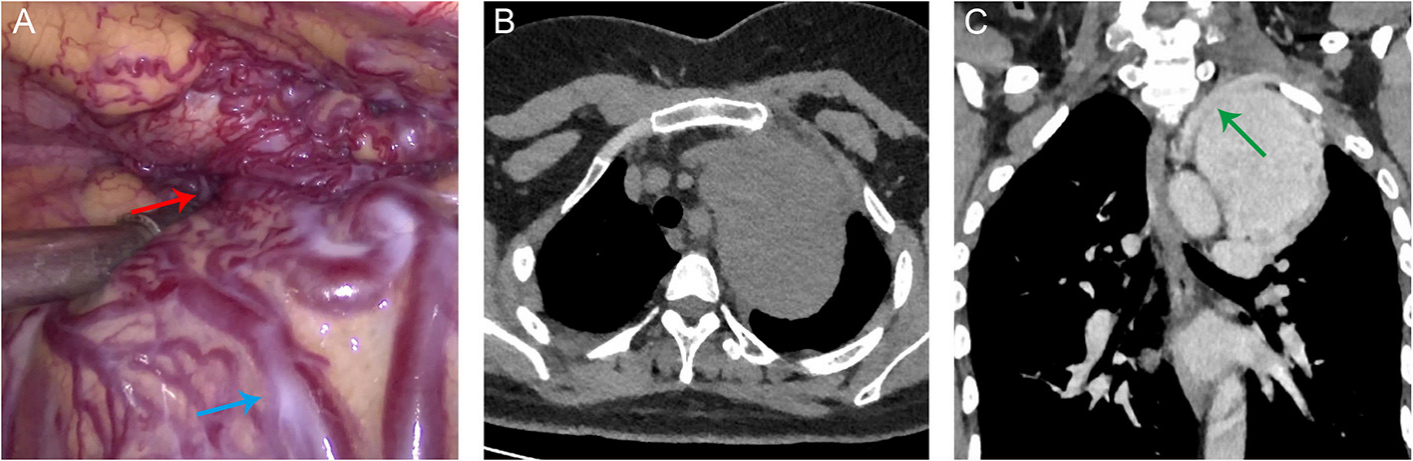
(A) Intraoperative photograph showing abundant tortuous blood vessels on the surface of the tumor (blue arrow) and an unclear border at the top of the chest (red arrow). (B) A CT scan revealed a giant anterosuperior mediastinal tumor (6.7 × 9.8 × 9.7 cm) near the left side of the aortic arch. (C) Contrast-enhanced CT scan showed that the tumor was surrounded by tortuous vessels and was poorly demarcated from the left subclavian artery (green arrow).
